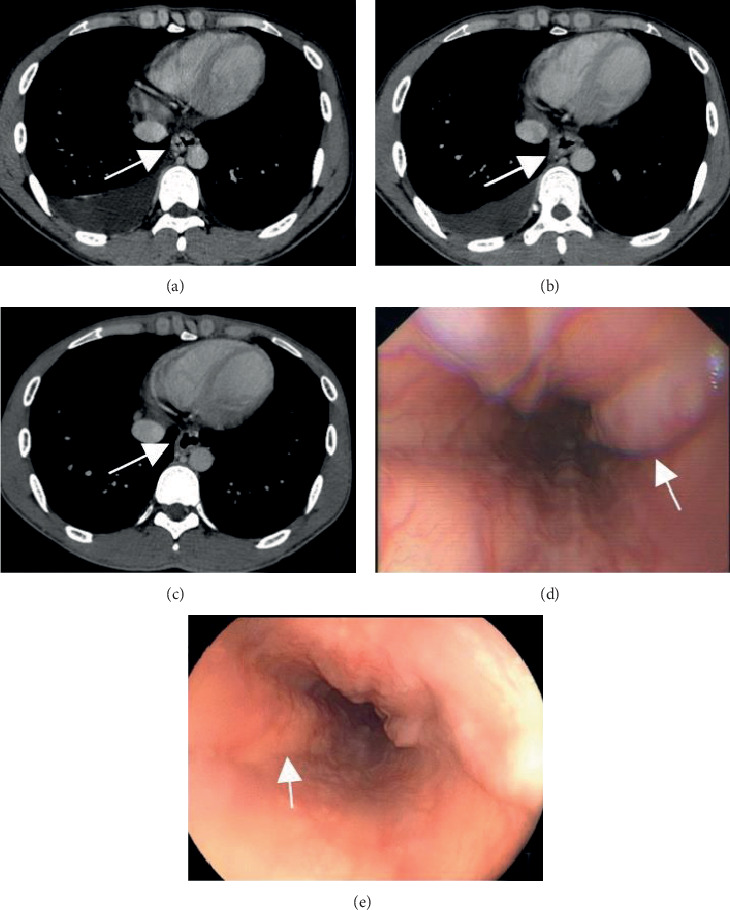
Efficacy of endotherapy in a patient with severe esophageal varices. Axial computed tomography images show that the (a) esophageal varices are attenuated with endoscopic band ligation at (b) 1 week and (c) 1 month after treatment. The (d) dilated varices are reduced at (e) 1 month after treatment on endoscopy.
